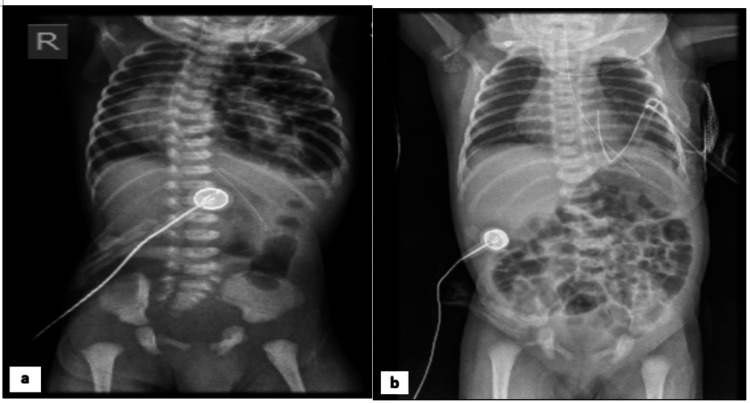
(a) Chest x ray showing herniation of the bowel into the left hemithorax; (b) Postoperative chest x-ray showing complete expansion of the left lung
